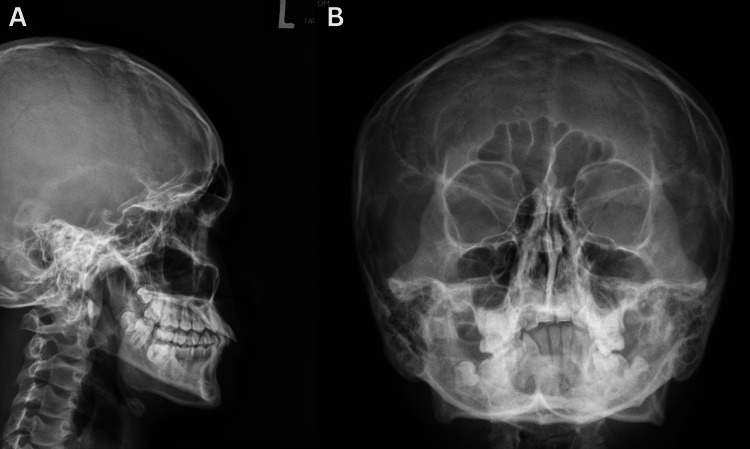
Skull radiographs showing no obvious orbital wall fracture: (A) lateral skull radiograph and (B) occipitomental (Waters view) radiograph
